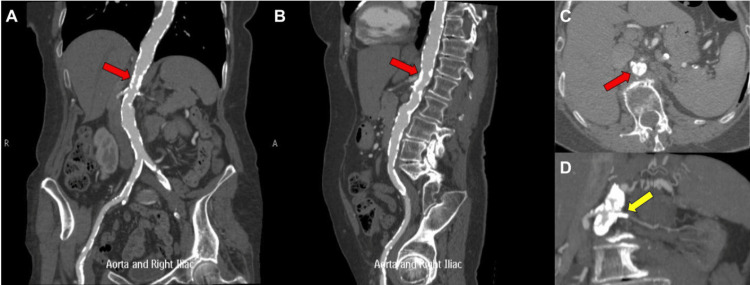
CT angiography of the abdominal aorta (A) Coronal, (B) sagittal, and (C) transverse computed tomography angiography images demonstrate prominent suprarenal intraluminal calcifications measuring approximately 3 mm (red arrow). (D) Coronal computed tomography angiography image shows complete occlusion of the left renal artery (yellow arrow). CT: computed tomography
